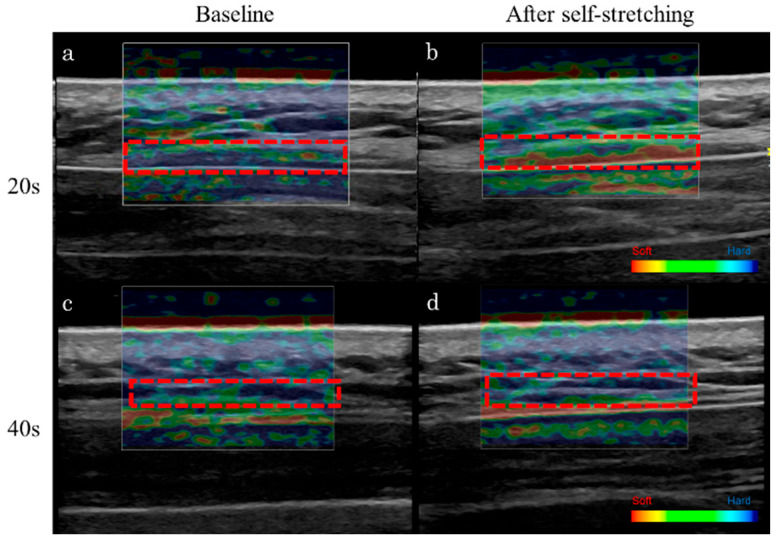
Changes in the strain ratio of the upper trapezius muscle after self-stretching assessed using ultrasound strain elastography. The 20-year-old participant exhibited a decrease in muscle stiffness following self-stretching (b) compared with baseline (a). However, the 40-year-old participant showed minimal change in muscle stiffness following self-stretching (d) compared with baseline (c). Red indicates softer tissue, whereas blue indicates harder tissue.
